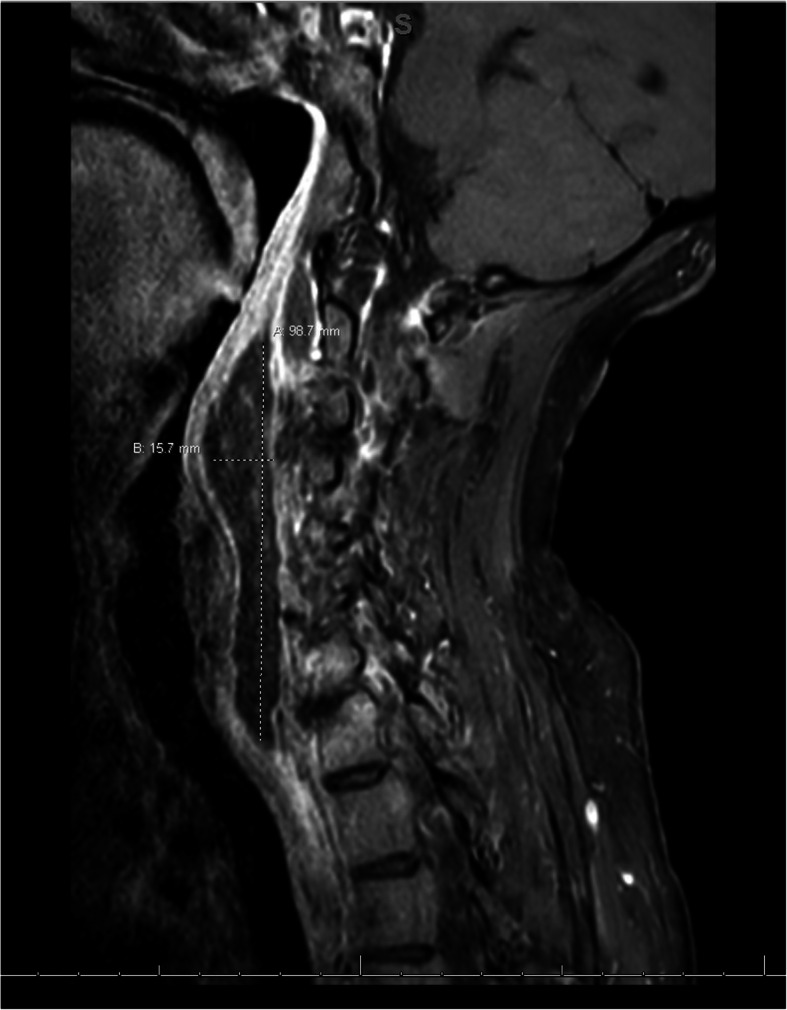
Sagittal MRI T1 fat suppression protocol with contrast, performed on admission (pre-operatively)
